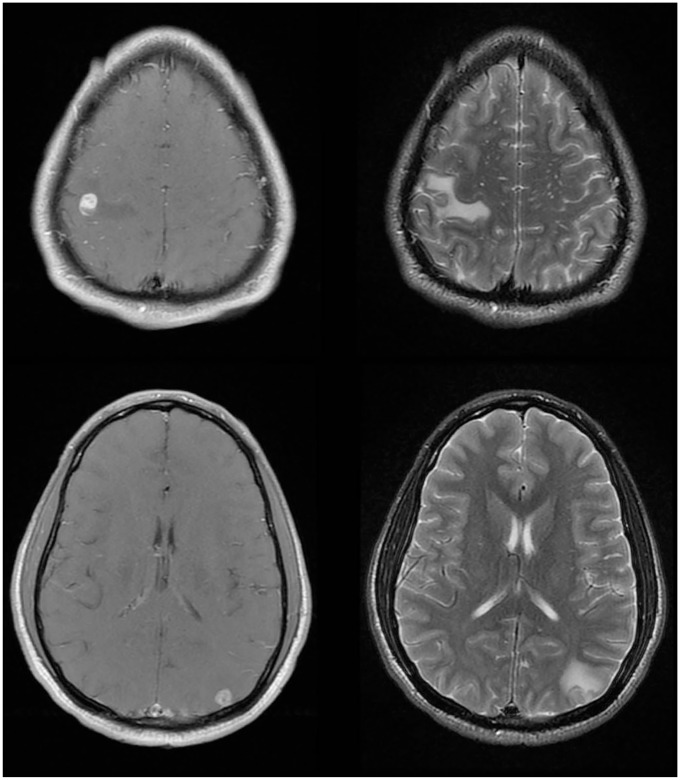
Magnetic resonance imaging of the brain demonstrates 2 enhancing masses, both
